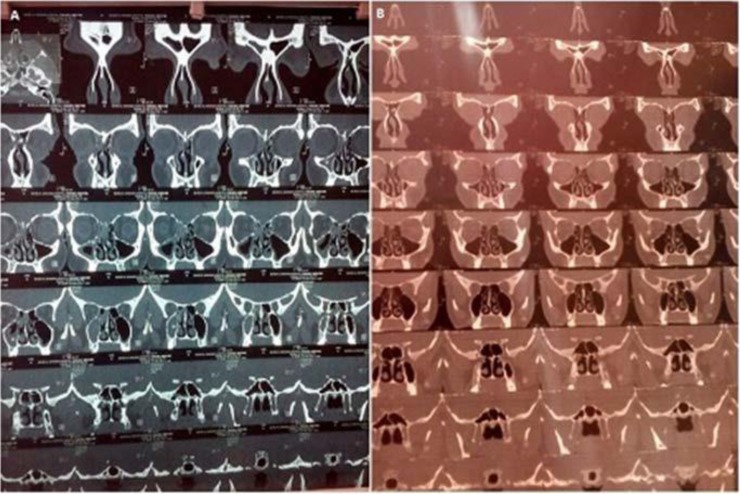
CT scan findings before (A) and after (B) the treatment
